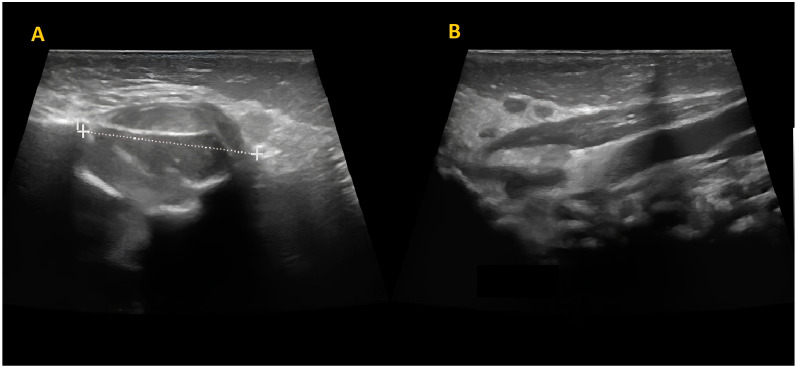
Skin and subcutaneous ultrasound. A fusiform mass over the left masseter muscle, inhomogeneous and hypoechogenic, sized 17 mm × 15 mm (A). Concurrent thickening of the left sternocleidomastoid muscle at the mastoid head with increased thickness compared to the contralateral side (8 mm) (B).
